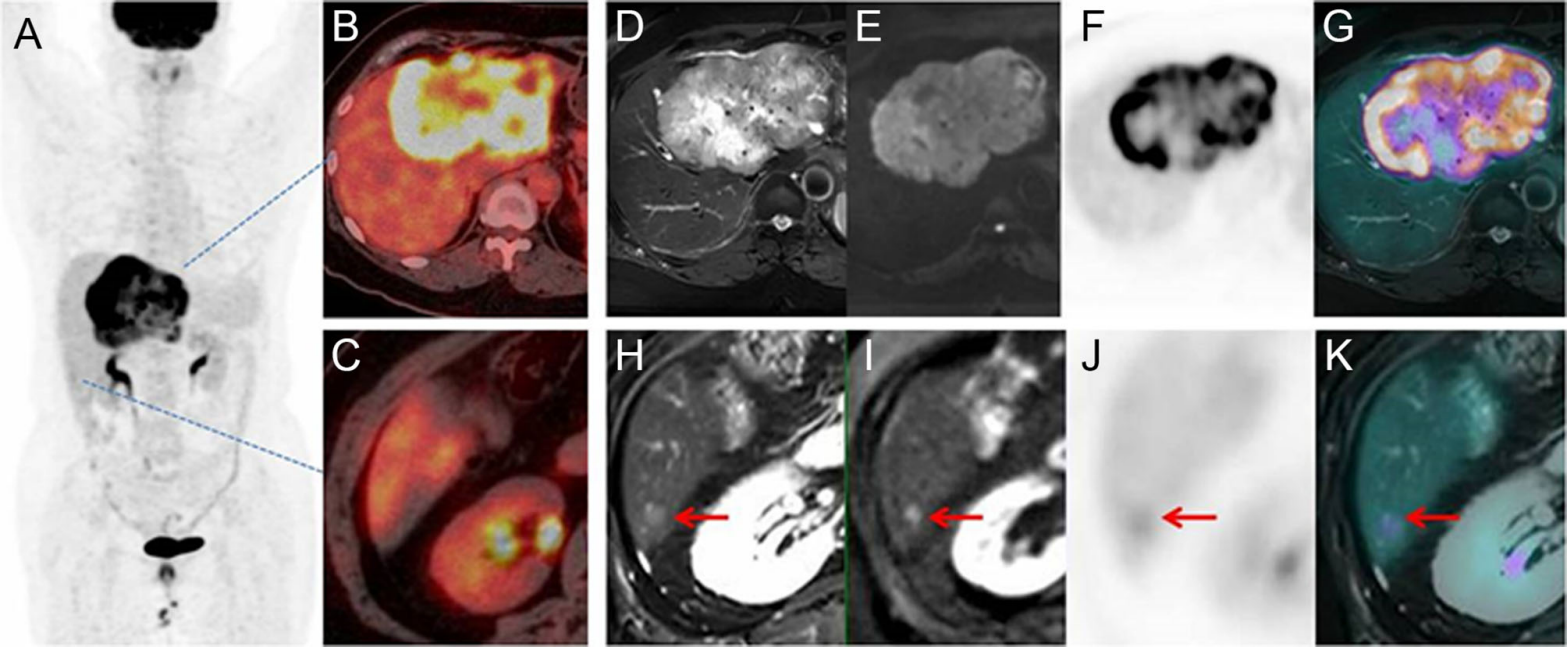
Images of a 63-year-old patient with liver mass. PET/CT MIP (A) and fusion image (B, C) showed a mass with high FDG uptake and no metastasis on the liver or distant organ. On PET/MR, the mass had hyperintensity on T2WI and DWI, with high FDG uptake on PET and fused image (D–G). A 6-mm lesion was an additional finding by PET/MR (H–K). Therefore, the stage was upgraded from M0 to M1.
